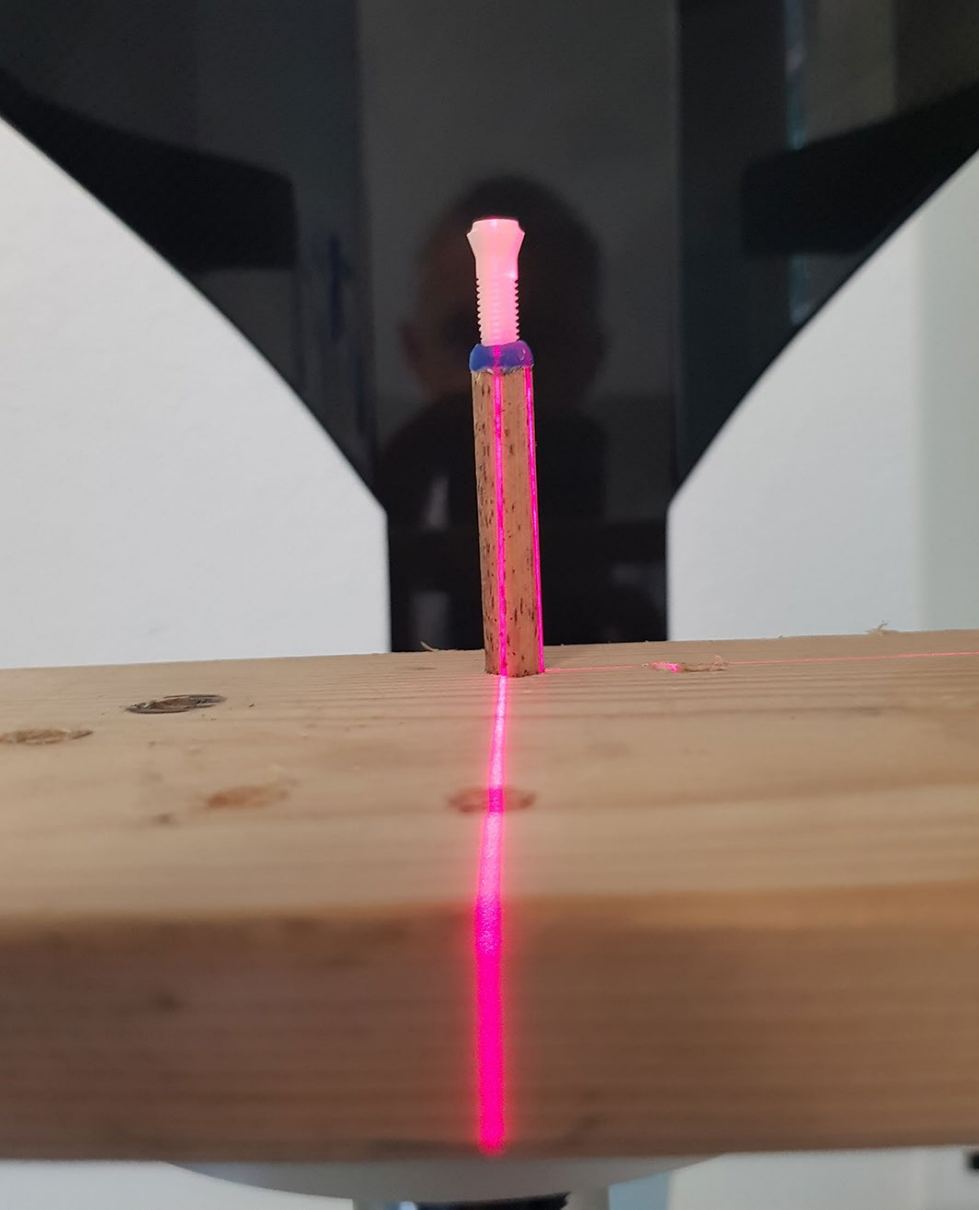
Set-up with the Y-TZP-implant centered and exposed in the CBCT-device. By means of the integrated laser-beam the central-ray was exactly centered in the field of view so that the required implant cross-section was centered in each PROJ.
